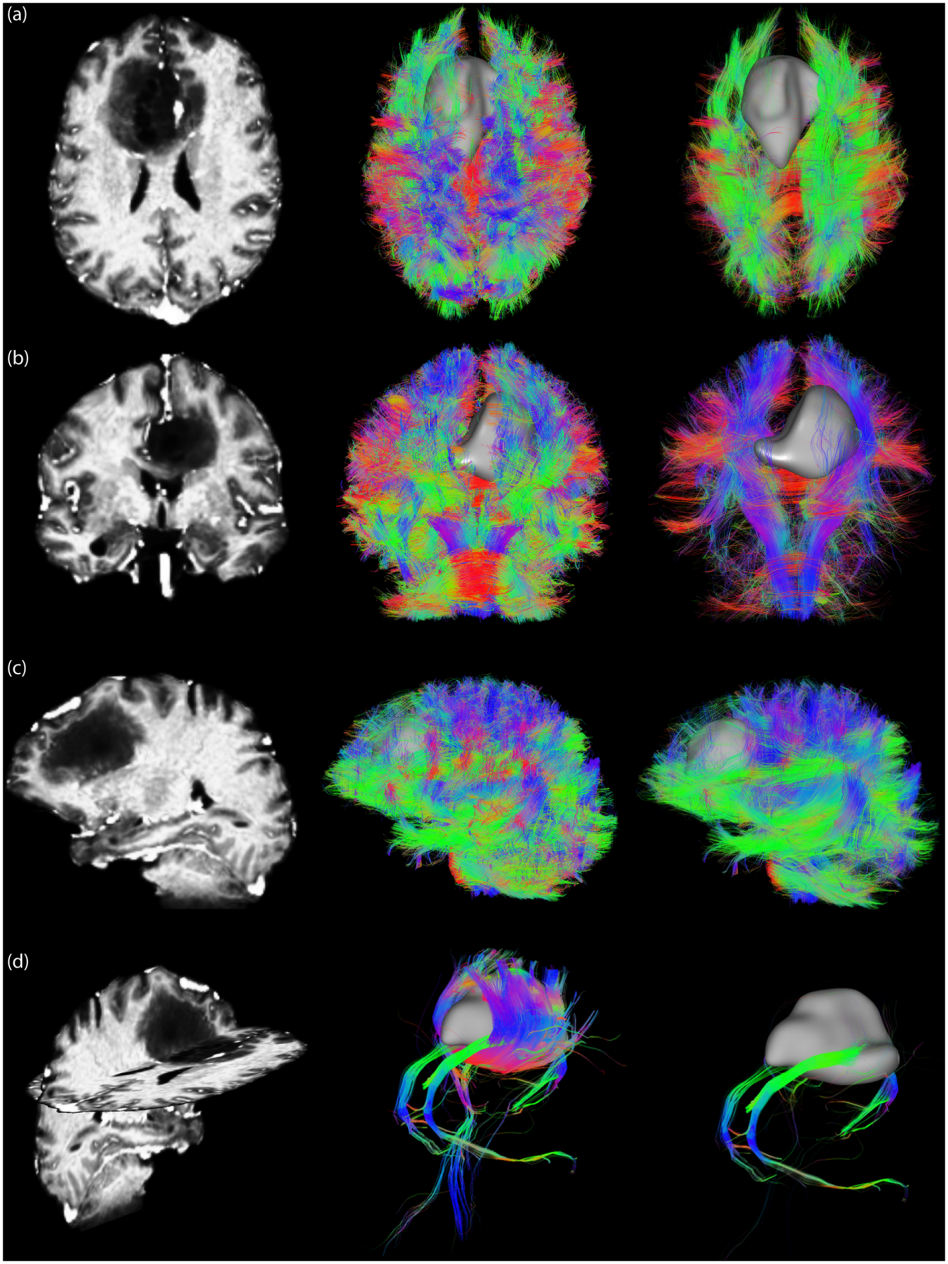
Neurosurgical application of the orientation-dependent transparency rendering method. The left column shows a high resolution T1-weighted image for anatomical reference. The middle column shows conventional streamline rendering, where the tumor (gray) is largely covered by the massive amount of streamlines. The right column shows that orientation-dependent transparency rendering (*α*
